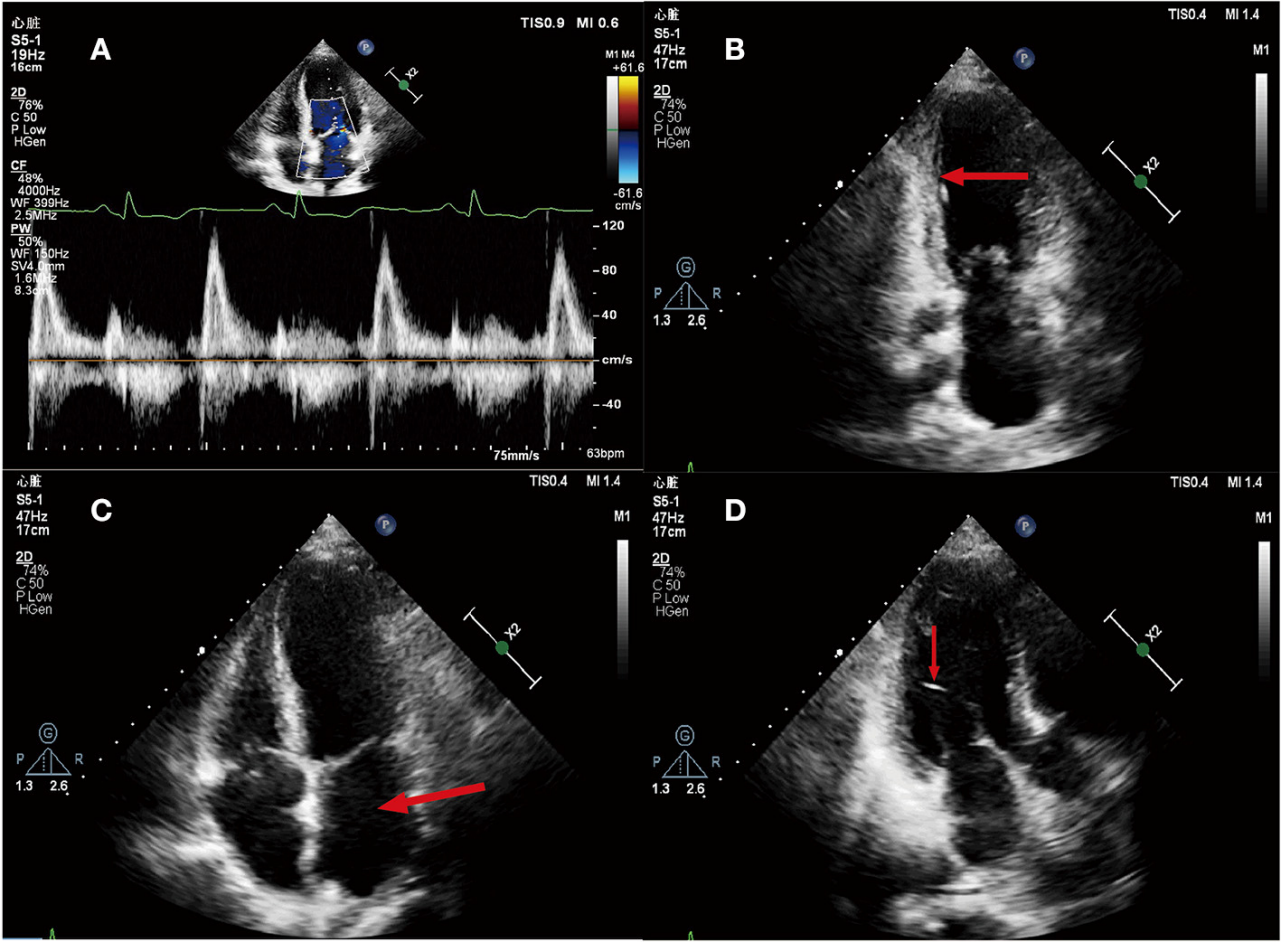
(A) Mitral flow spectrum Doppler; (B) Apical two-chamber heart view, Ventricular systole. The arrow shows that the inferior wall of left ventricle became thinner, the echo increased, and the mobility decreased; (C) Apical four-chamber heart view. The arrow indicates the enlargement of left atrial; (D) Apical two-chamber heart view, Ventricular systole. The arrow shows a left ventricular false tendon.
